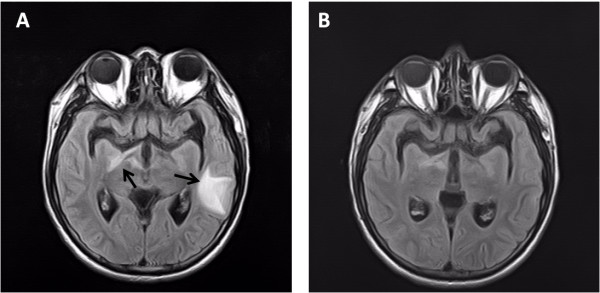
Brain MRI of the patient after cessation of corticosteroid. Axial T2-FLAIR images showed new cerebral lesions (arrows in A). After 16 days on intensified anti-cryptococcal therapy, T2-FLAIR demonstrated near complete resolution of cerebral lesions (B).
